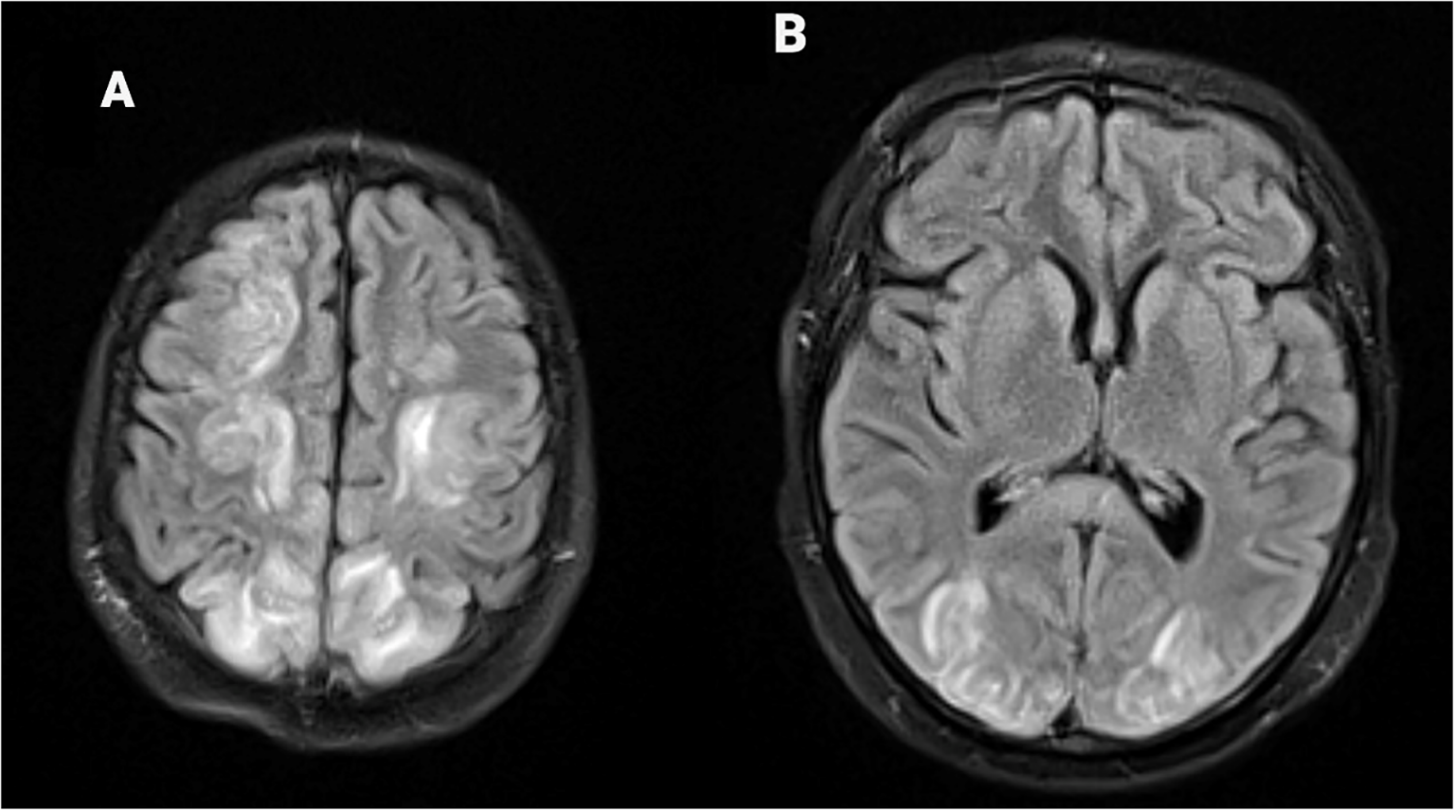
Axial MR brain (A, B) FLAIR demonstrates bilateral symmetrical widespread cortical/subcortical T2/FLAIR with high signal intensity, suggesting vasogenic edema in the frontal, parietal region (A) and occipital lobes (B).
